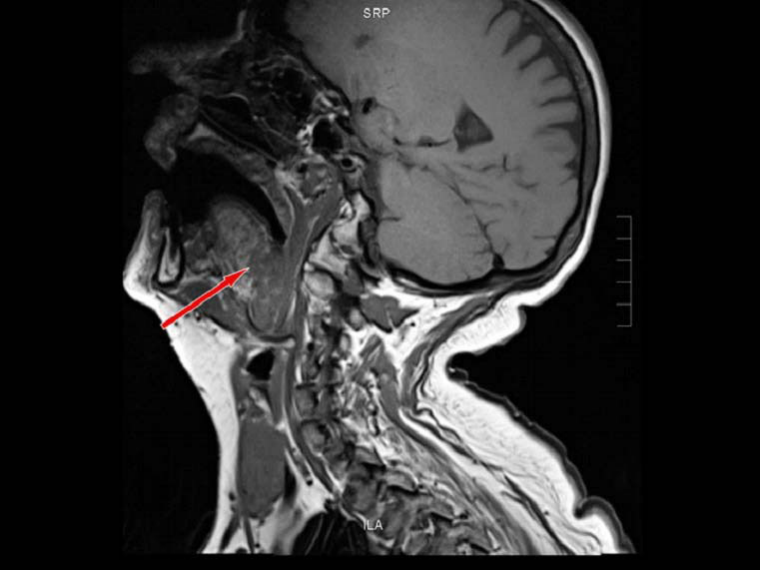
Axial T2 Fat saturated MRI representing mass invading right base of tongue extending across the midline involving the right faucial tonsil. Mass shows immediate T2 signal enhancement with bright focus within.
